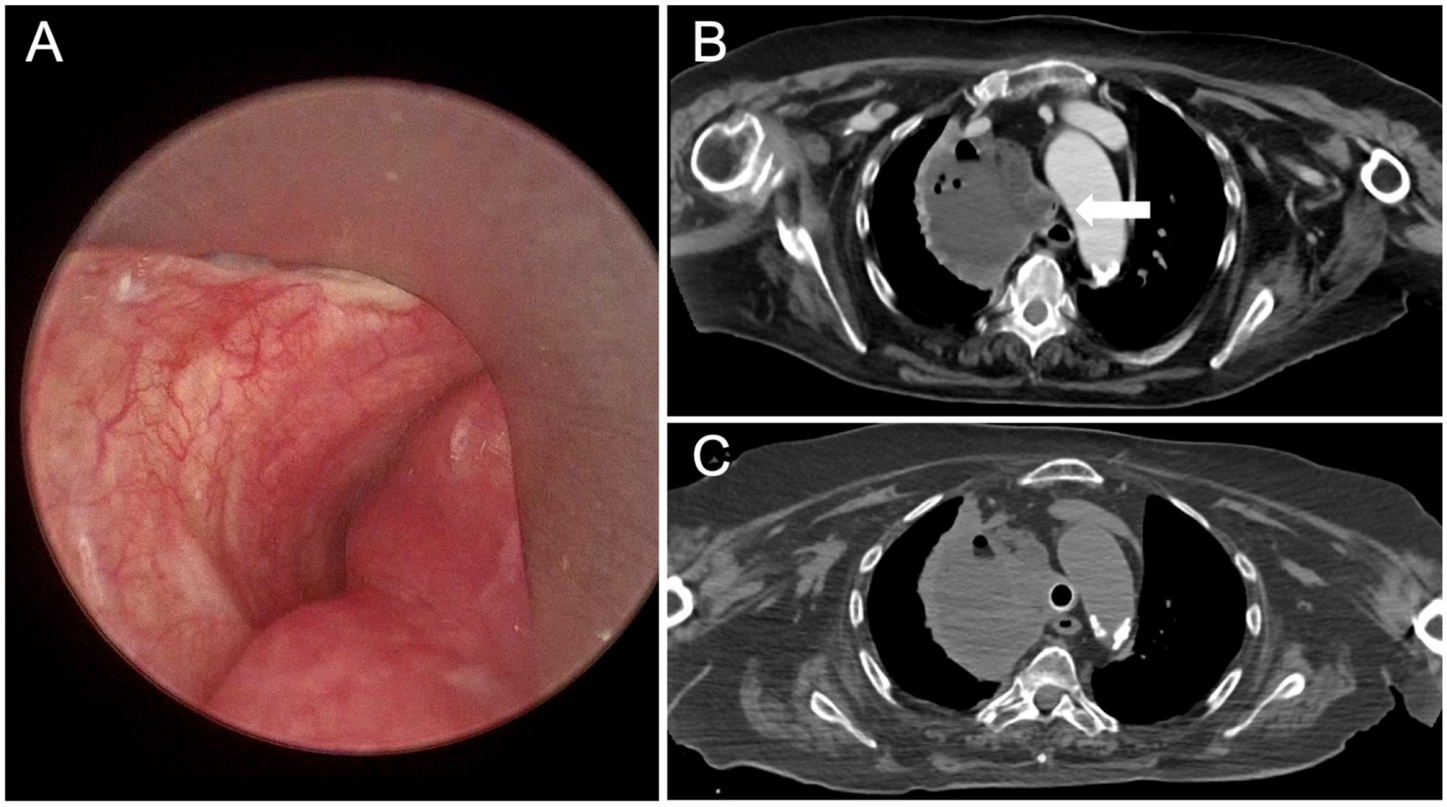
Benign CAO in patient with lung abscess and tracheal compression. (A) Bronchoscopic view. (B) Chest CT scan showing the axial plan of a trachea with severe stenosis. (C) Chest CT axial scan after rigid bronchoscopy, tracheal dilation and stent placement.
